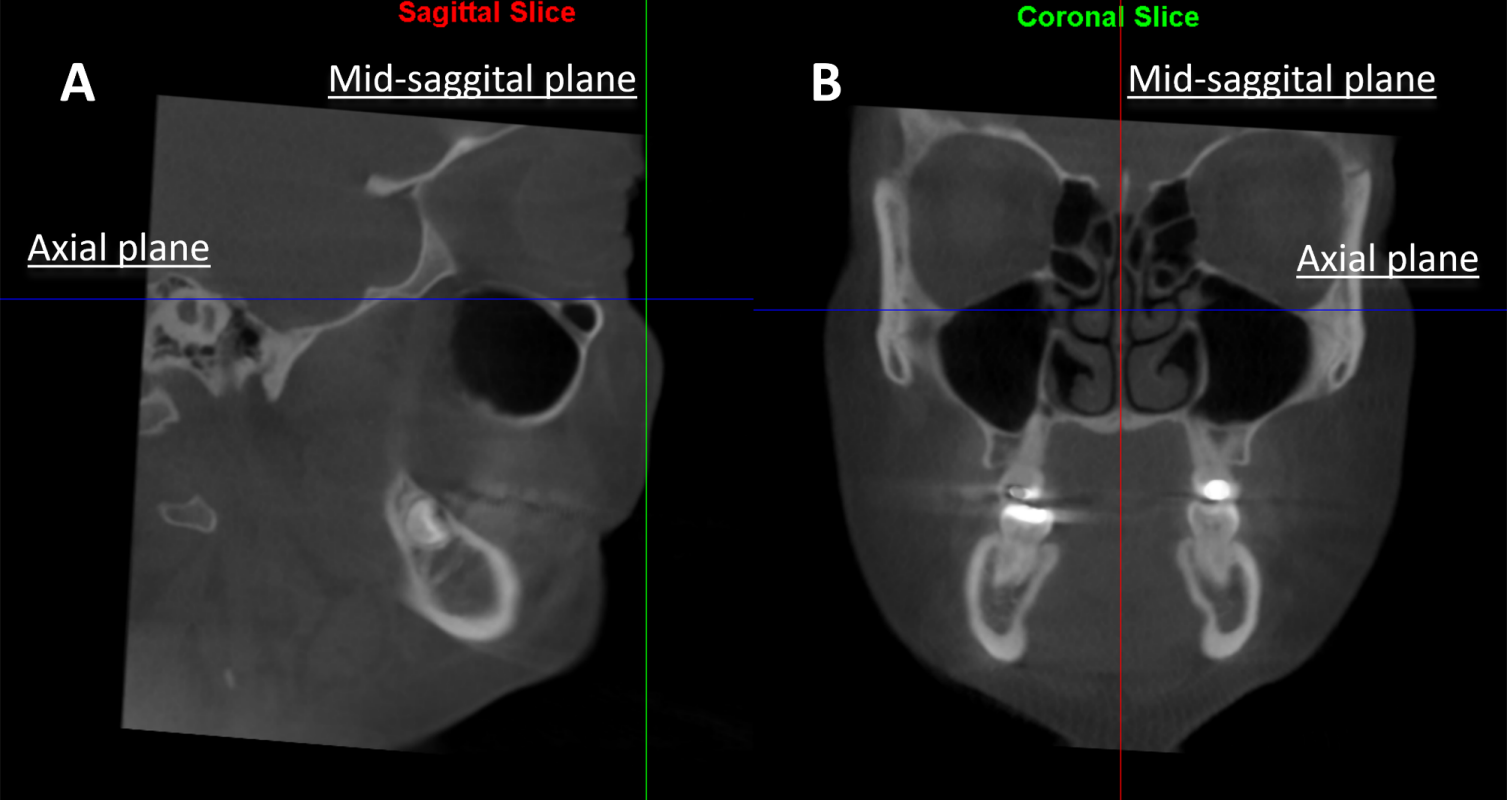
CBCT images were oriented in the sagittal view with coronal plane aligned with Nasion perpendicular and axial plane passing through FHP (A) and in the coronal plane with mid-sagittal plane aligned with Nasion perpendicular and axial plane passing through the infraorbital rim (B)
